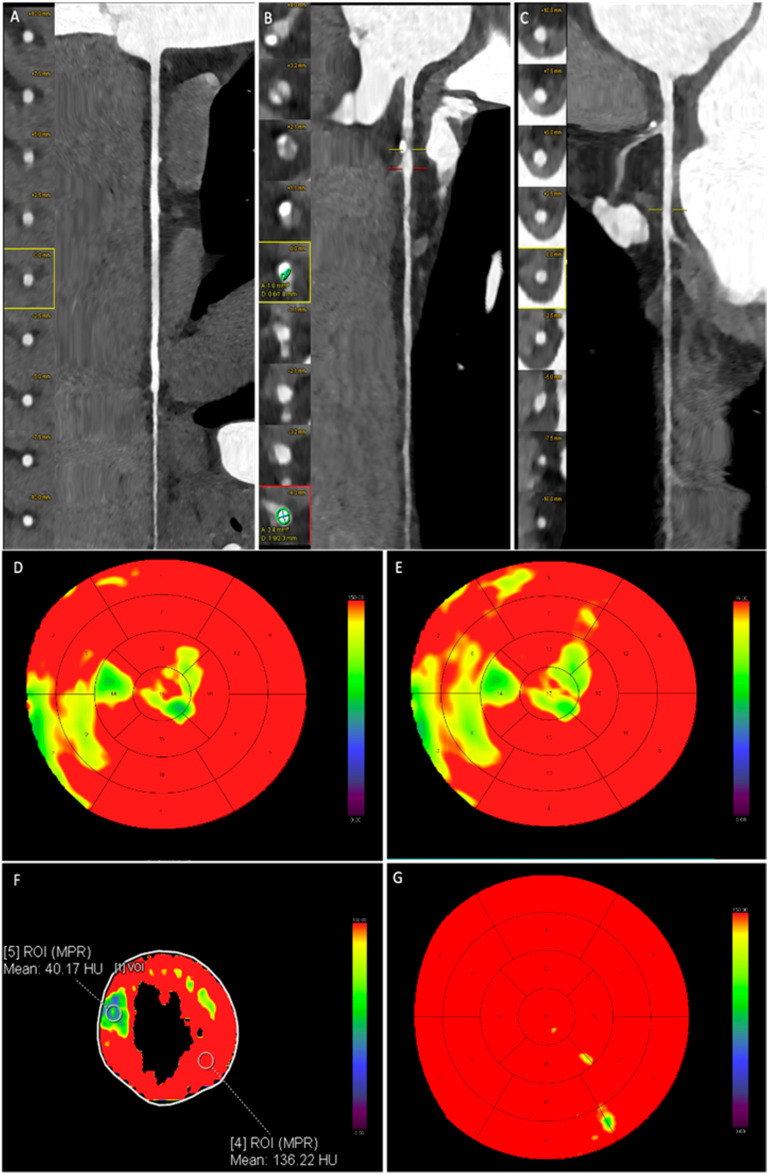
Male patient, 61 years old, with atypical chest pain. Coronary CT angiography reveals a regularly perfused right coronary artery without significant stenosis (A). The left anterior descending artery shows a single moderate stenosis with diameter reduction between 50 and 69%, located on a high-risk plaque at the proximal segment (B). The circumflex artery is regularly perfused and free from significant stenosis (C). Dynamic stress CT myocardial perfusion imaging highlights reduced myocardial perfusion in some basal, mid, and apical septal segments ((D,E), yellow-green area). In the subsequent post-processing (F), the reduction in blood flow is quantitatively assessed by placing a region of interest (ROI) in hypoperfused segments and compared with reference segments. (G) shows the normal myocardial vascularization during the baseline evaluation of CCTA.
